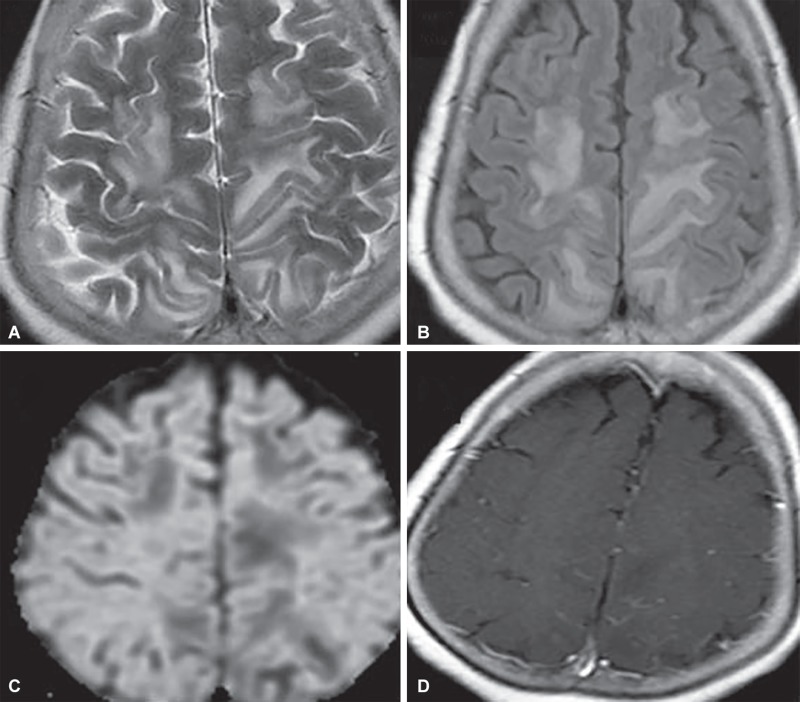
Posterior reversible encephalopathy syndrome. Symmetrical T2W (A) and FLAIR (B) hyperintensity in parieto-occipital subcortical white matter. No diffusion restriction (C) or contrast enhancement (D) helps to differentiate from infarctions
